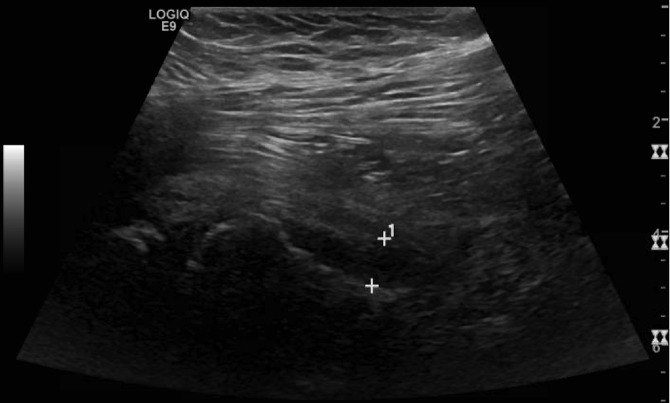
Ultrasonography at time of presentation showing right hip effusion.
